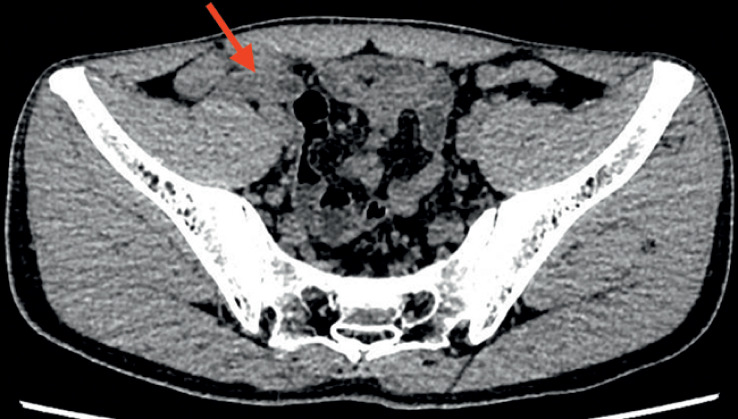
Computed tomography scan image showing acute suppurative appendicitis, with an enlarged appendix (arrow).
